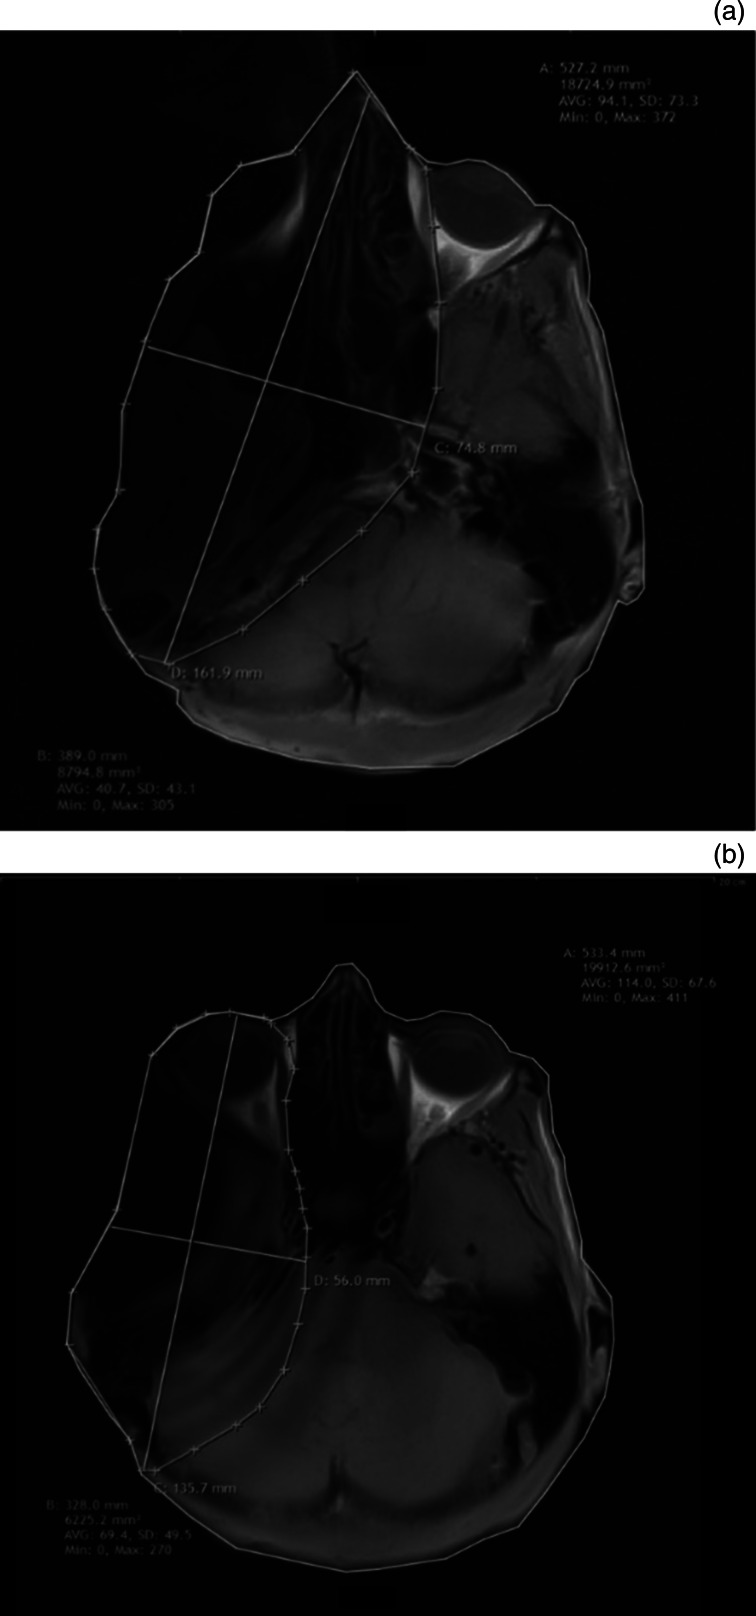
T1-weighted axial magnetic resonance imaging scans showing: (a) image without reduction sequences and (b) image with slice-encoding metal artifact correction view-angle tilting metal artifact reduction technique sequences applied. *A = measurement for the full head; B = measurement taken for area of the implant;* C/D = measurement taken for width; AVG = average; SD = standard deviation; Min = minimum; Max = maximum
